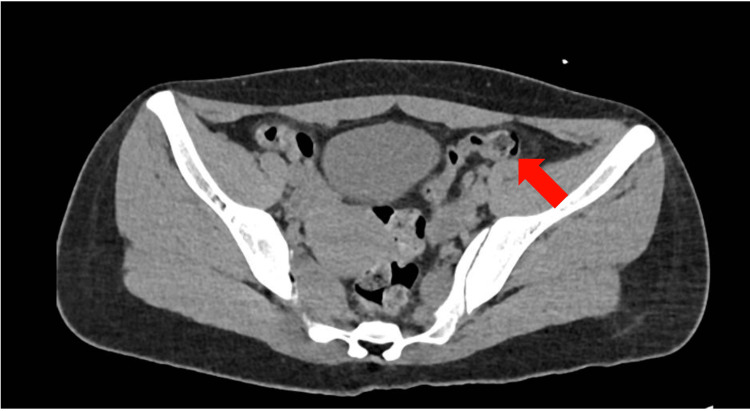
Abdominal CT. Abdominal CT scan revealing enlargement of the left adnexa (volume 11.6 cc) and increased sacrococcygeal angle.
